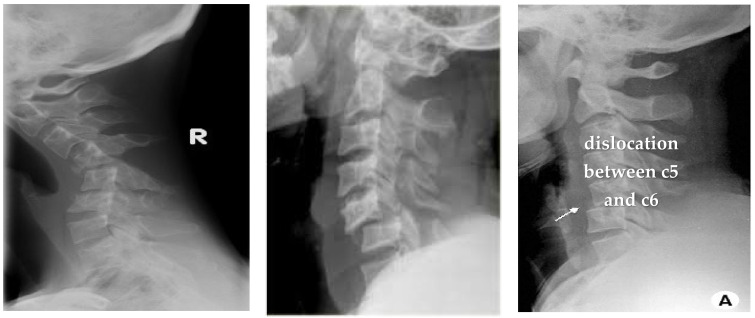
X-ray images for the dislocated CS.
